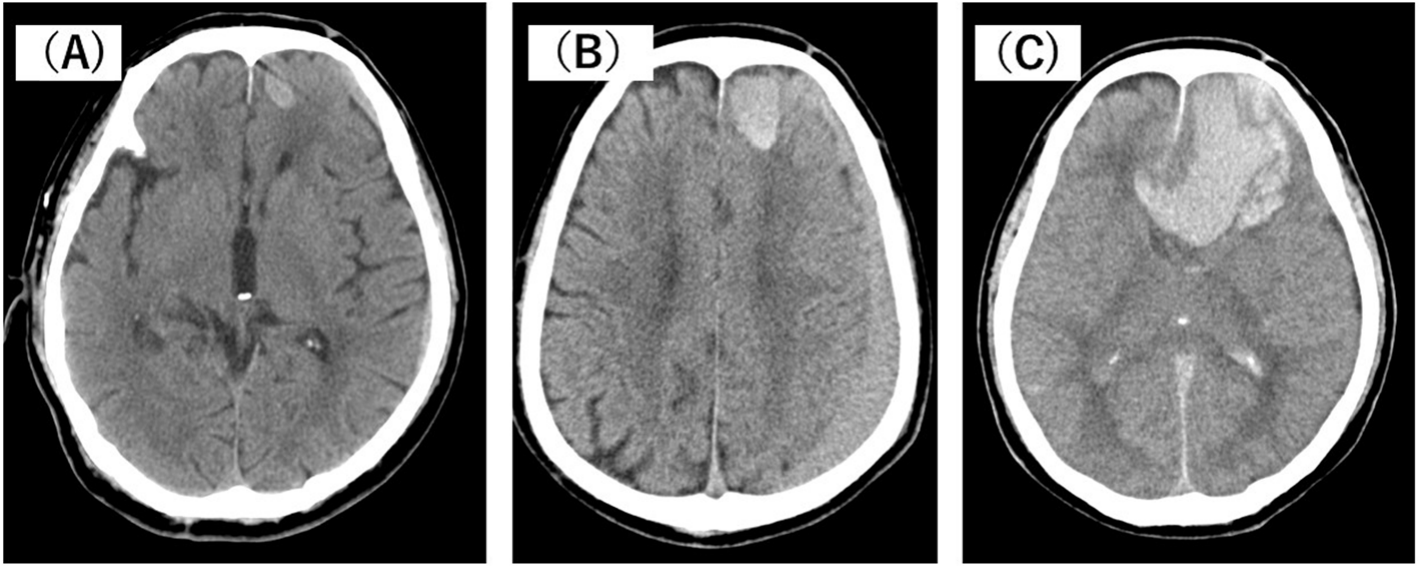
The clinical course of intracranial lesions in the head computed tomography (CT). A Head CT immediately after the head injury showed cerebral contusion in the left frontal lobe and a left subacute subdural hematoma (performed at a previous hospital). B Two hours after the head injury, the left subacute subdural hematoma was not significantly changed, but the brain contusion worsened. C Seven hours after the head injury, the hematoma in the left frontal lobe was markedly enlarged and had perforated into the ventricle
